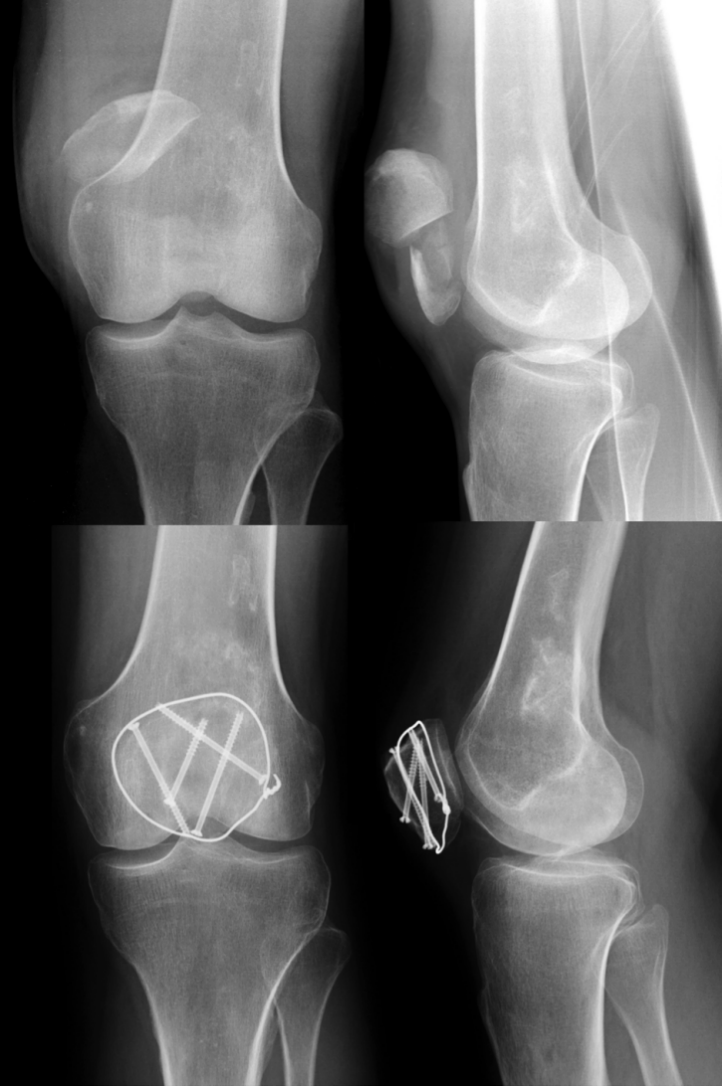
Preoperative X-rays of a comminuted patella fracture (above). 3 months post-surgery using screw fixation and modified tension band (below).
